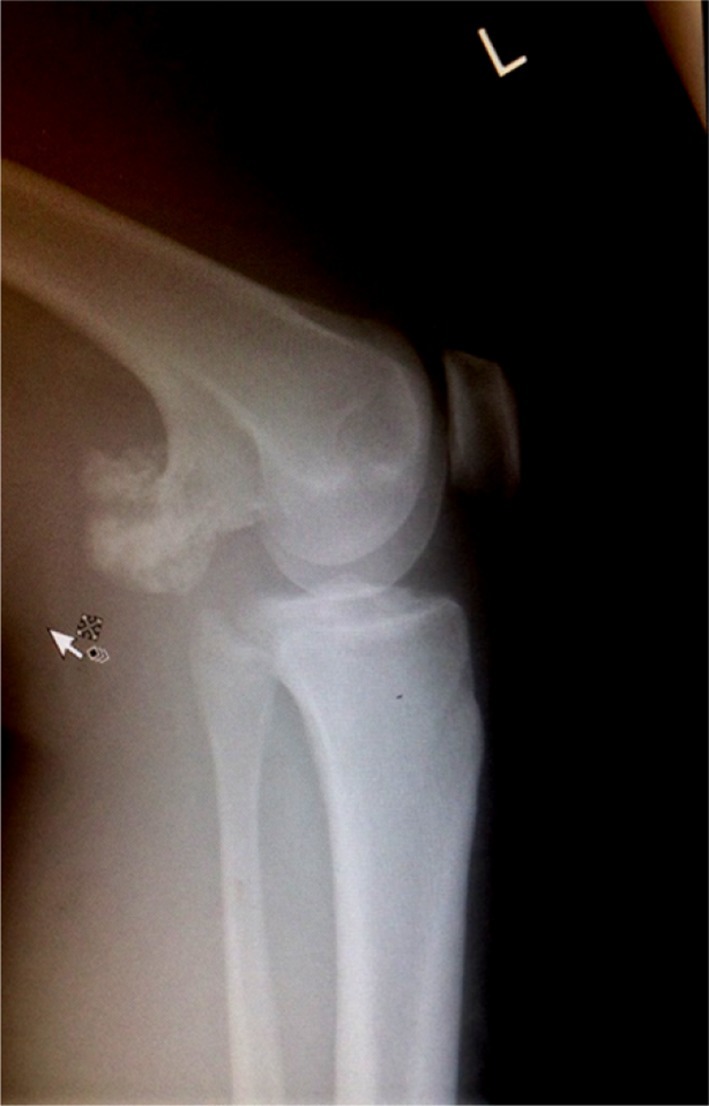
X-ray imaging revealed a large bony growth originating from the distal femur in the popliteal fossa.
